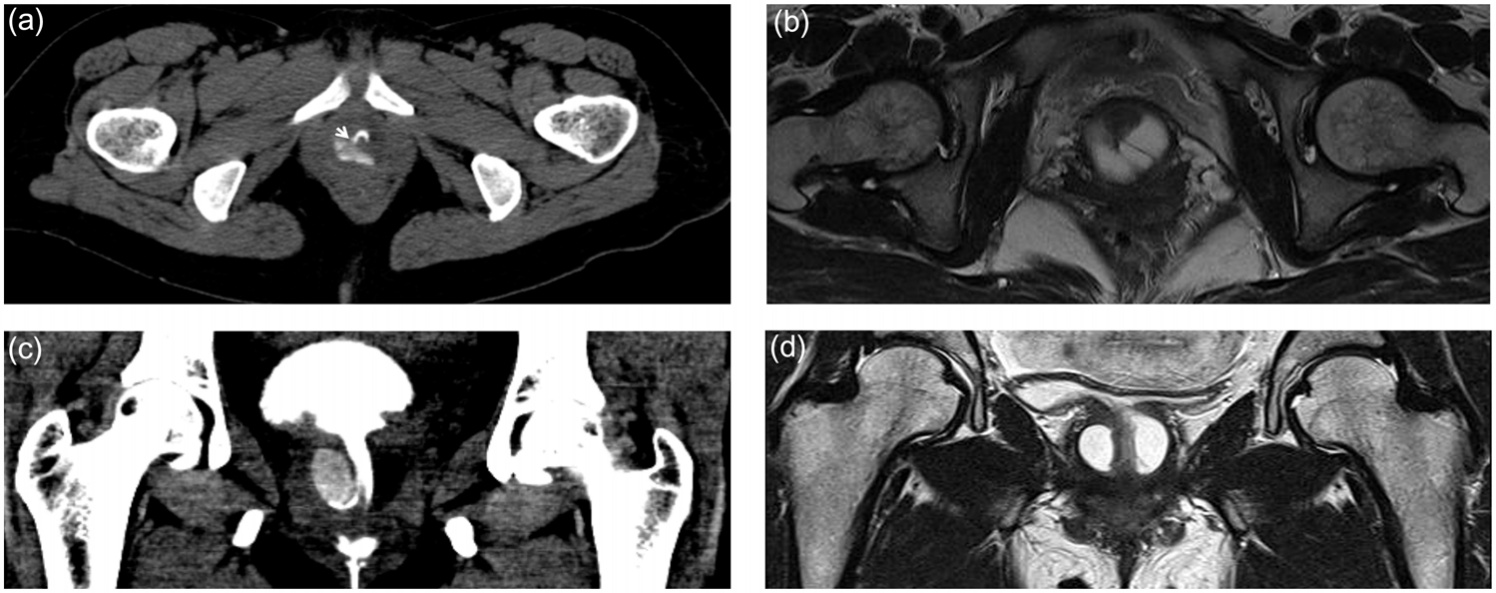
Comparison of CT-VCUG and MRI in a 42 year-old female. (a) The axial image of CT-VCUG of voiding phase showed a diverticulum partially filled with contrast media. (b) The axial image of T2 weighted MRI demonstrated the overall shape and complexity of the urethral diverticulum. (c) Coronal image of CT-VCUG revealed the ostium clearly. (d) Coronal image of T2 weighted MRI revealed suspicious ostium, but not as clearly as in CT-VCUG.
